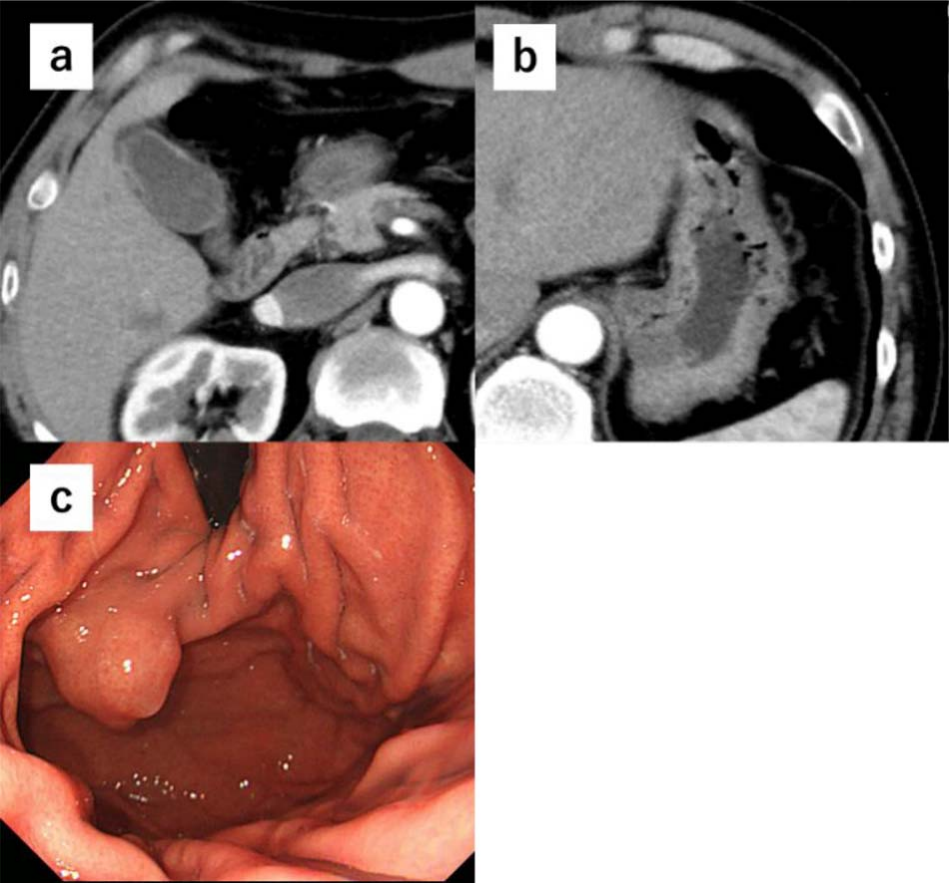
(a) CT shows cholecystitis, and no gall bladder is found. (b) CT shows cholecystitis, and no gall bladder was found. A submucosal tumor measuring 3.5 cm in diameter was incidentally detected. (c) Upper endoscopy reveals the tumor in the anterior wall of the gastric cardia near the EGJ.
